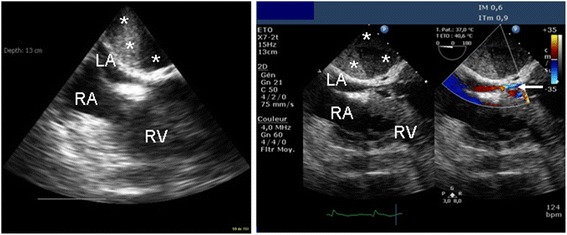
Extrapericardial tamponade. The miniaturized transesophageal echocardiographic probe disclosed a large mediastinal hematoma compressing the left atrium in this patient with severe shock (*left panel, asterisks*). The presence of a localized tamponade was confirmed during conventional transesophageal echocardiography which depicted a rounded heterogeneous mass consistent with a recent hematoma which compressed the left atrium and impaired atrial filling (*mid panel, asterisks*), as reflected by a narrow and turbulent inflow on color Doppler mapping (*right panel, arrow*). Abbreviations: LA, left atrium; RA, right atrium; RV, right ventricle
